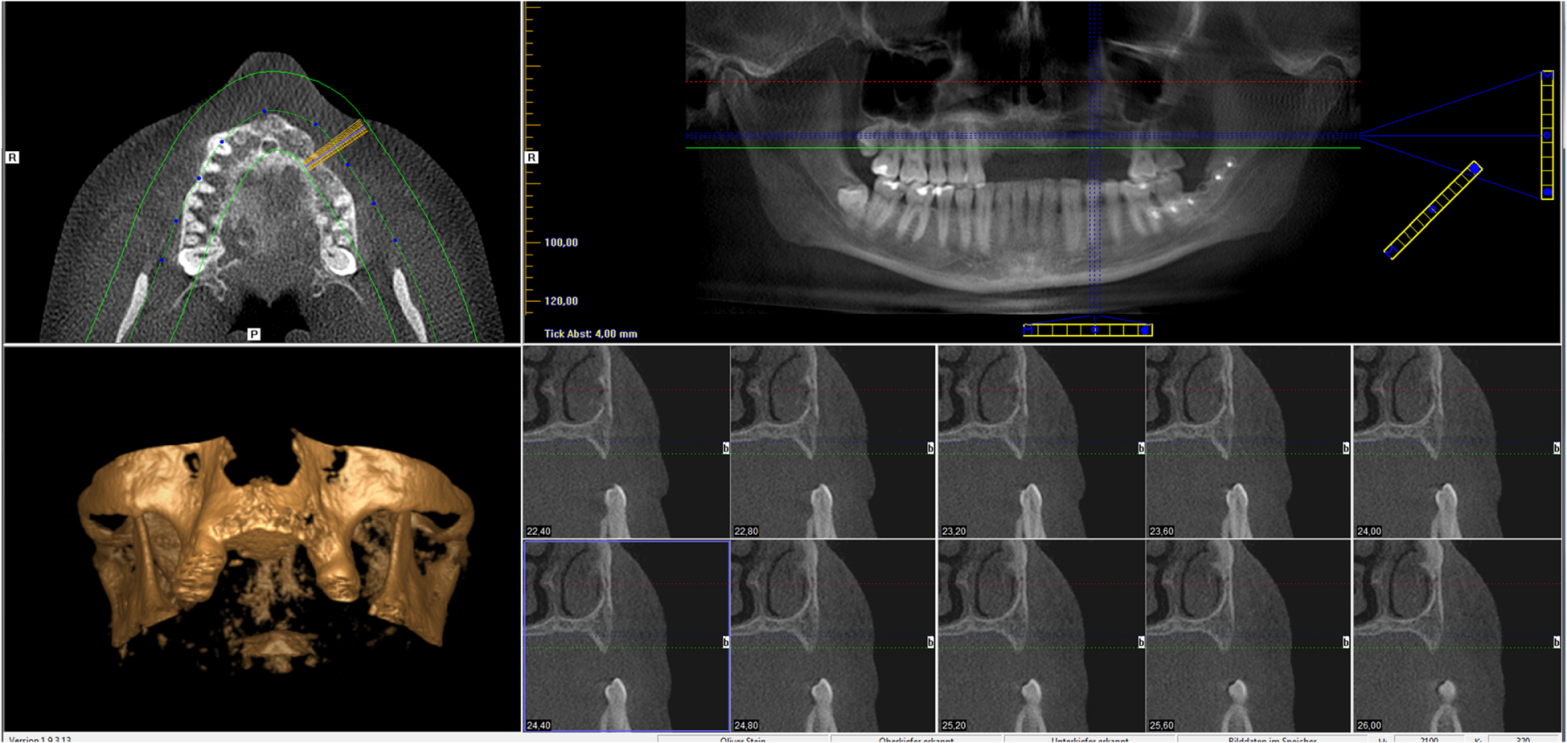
Preoperative CBCT scan showing the vertical and horizontal deficit
